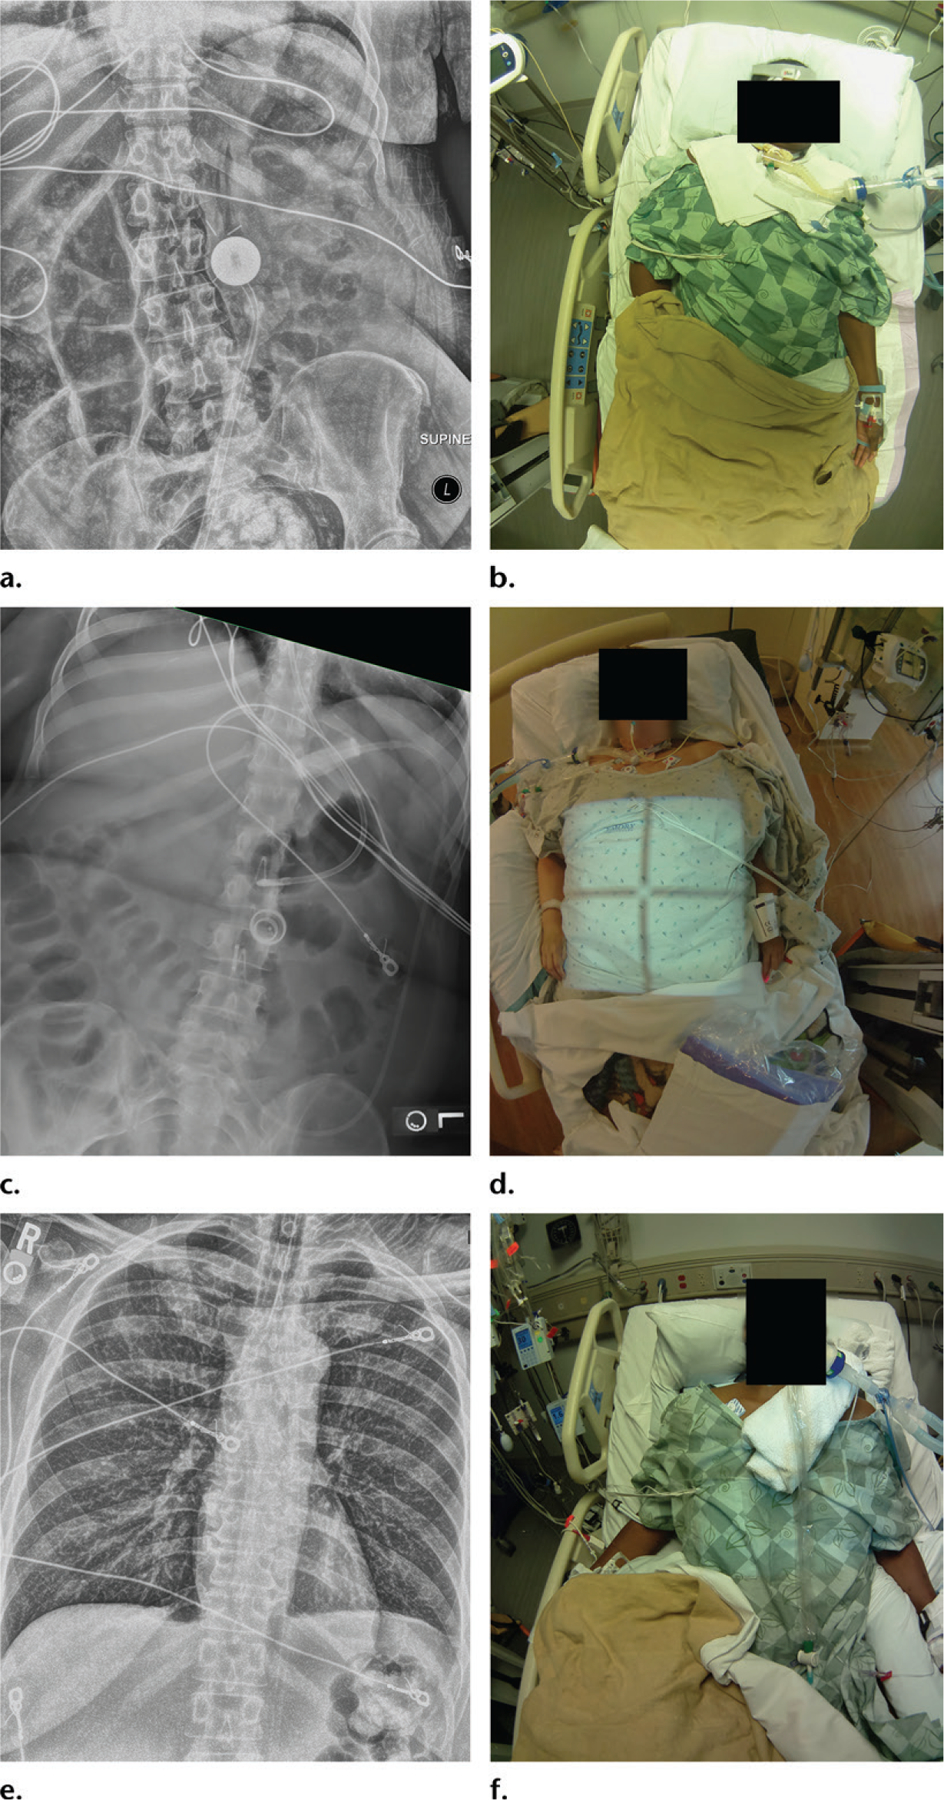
Wrong-patient error. (a, b) Portable abdominal radiograph (a) and photograph (b) were obtained in an African American woman with nausea and vomiting. (c, d) Portable radiograph (c) and photograph (d) obtained 6 days earlier and used as a comparison study show a different patient, a white woman with a gastric band. The differences between the photographs were noted by the interpreting radiology resident. (e, f) Portable radiograph (e) and photograph (f) from a more remote study obtained 8 days earlier show that the patient in c and d was the wrong patient. This error had not been noticed during the interpretation of c, as the interpreting radiologist had not looked at the corresponding photograph (d). The challenge was to determine the identity of the patient depicted in c and d. The technologist recognized the floor in the room where the study was performed and noticed some features in the background in d. The technologist took the photograph (d) to the floor, where a nurse was able to identify the patient.
